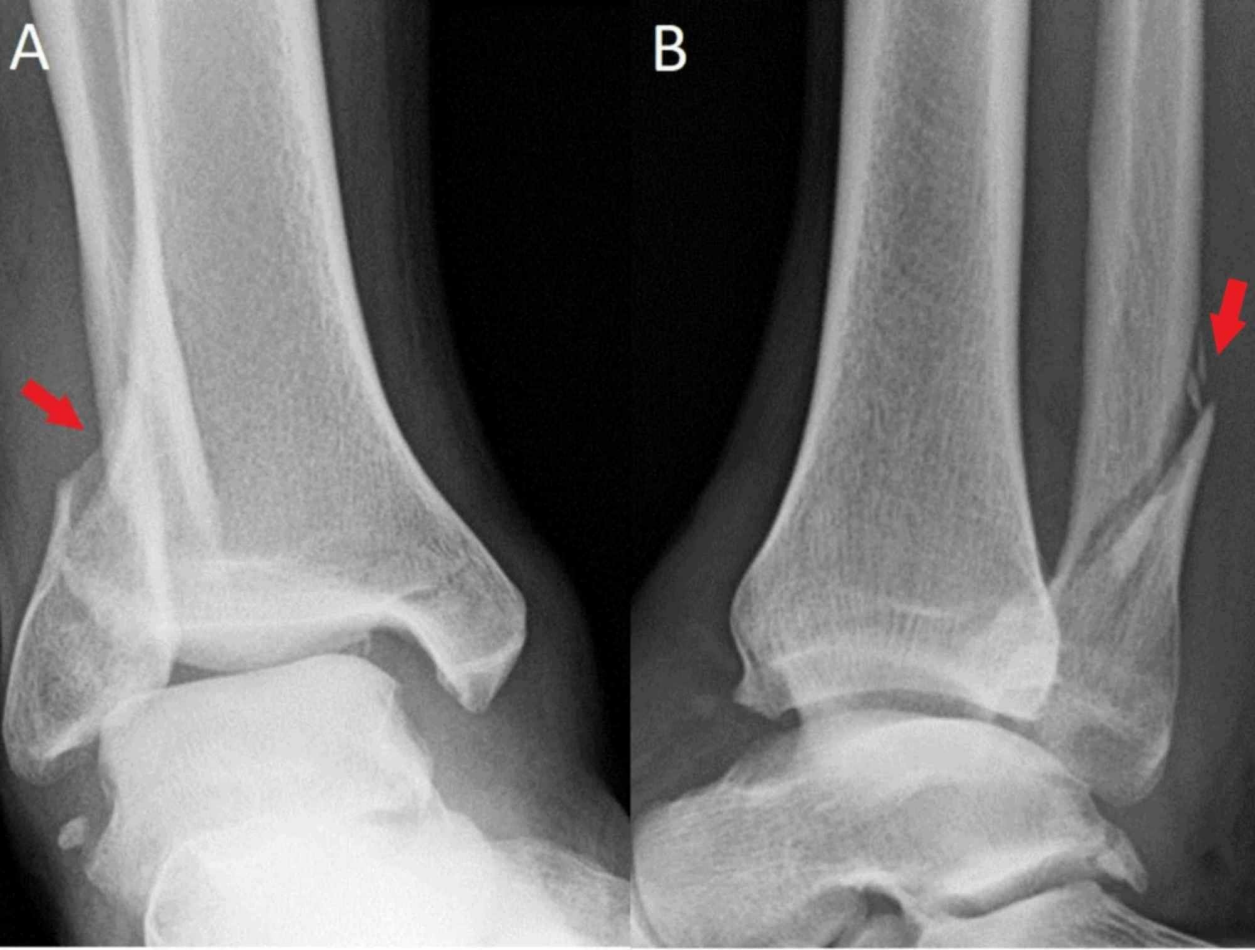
First attempted closed reduction Anteroposterior (A) and lateral (B) radiographs after first attempted closed reduction with notable unacceptable alignment and subluxation of the tibiotalar joint. These radiographs show clearly that the proximal fibular fragment is entrapped posterior to the tibia (red arrow).
